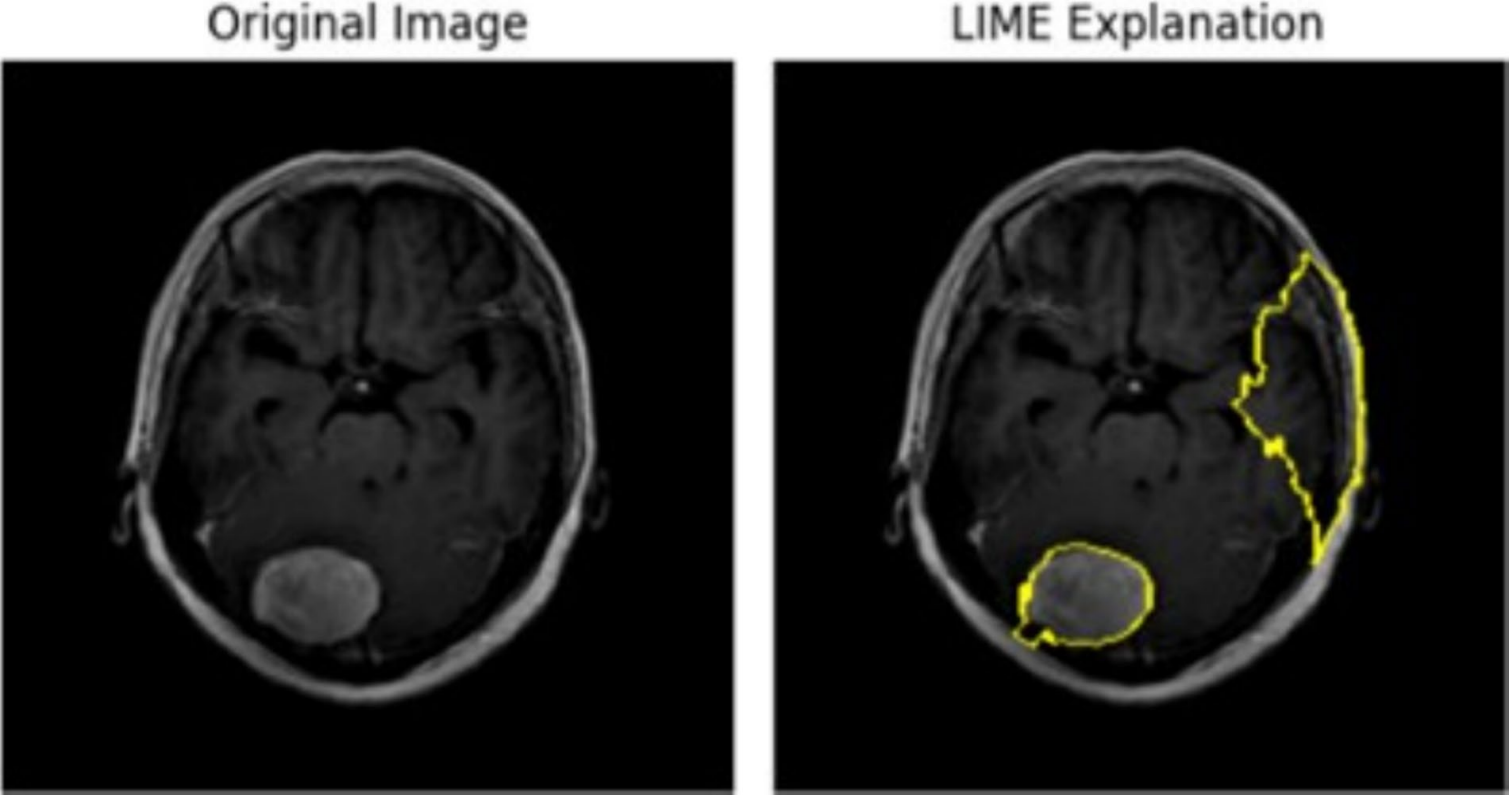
Meningioma tumor MRI image highlighting key areas of the scan when passed through Densenet50 model for classification.
